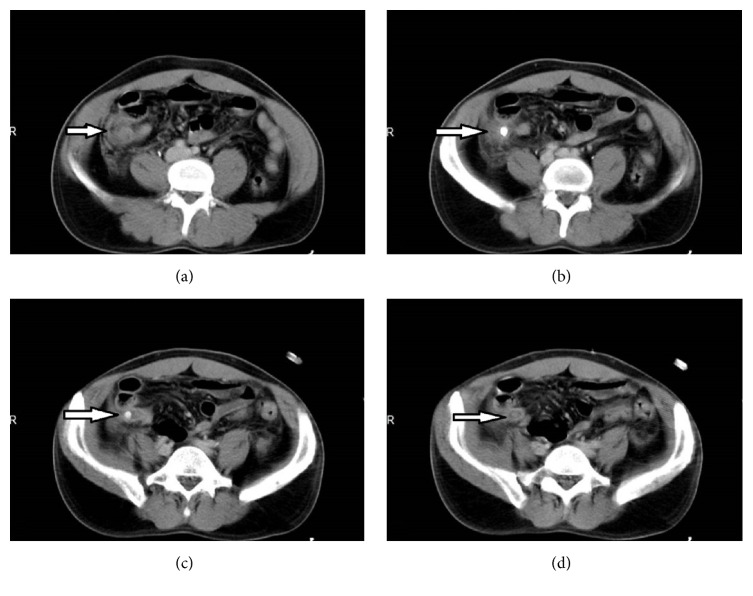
IV contrast enhanced axial abdominal CT images of a 43-year-old man with positive appendectomy. (a) Periappendiceal fat stranding and free fluid, (b) appendicolith in appendix lumen and periappendiceal free fluid, (c) appendicolith in dilated appendix lumen measuring 8 mm and periappendiceal fat stranding, and (d) wall thickening of dilated appendix.
